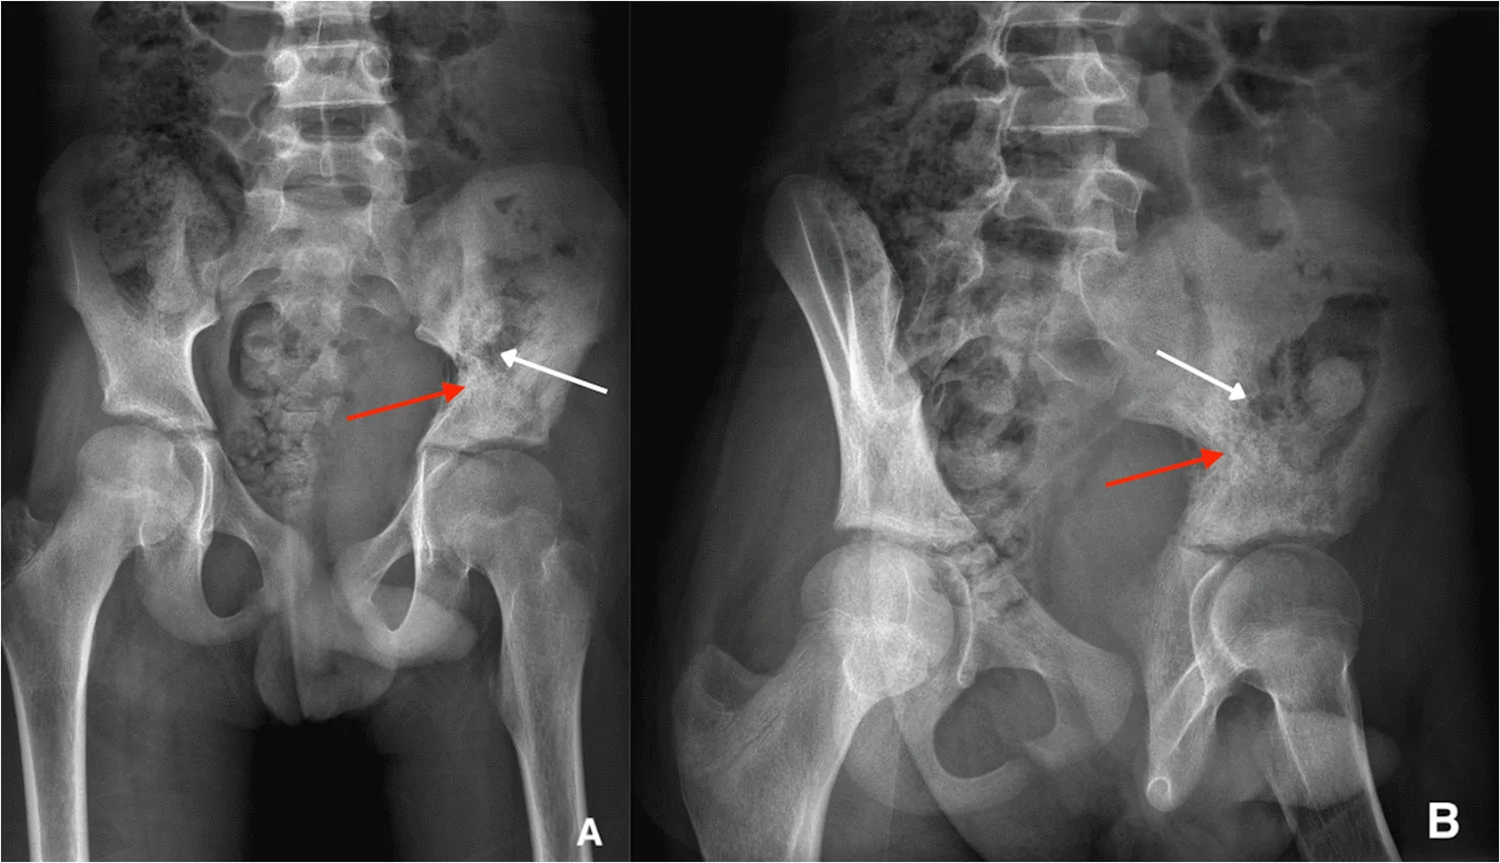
AP (A) and oblique (B) pelvis radiographs demonstrate an ill-defined osteolytic lesion in the left supra-acetabular ilium (white arrows) with prominent surrounding reactive sclerosis (red arrows) and a wide zone of transition. The combination of lysis and sclerosis suggests a relatively chronic or indolent osseous process despite its aggressive appearance Lesion conspicuity is somewhat limited by overlying bowel loops
